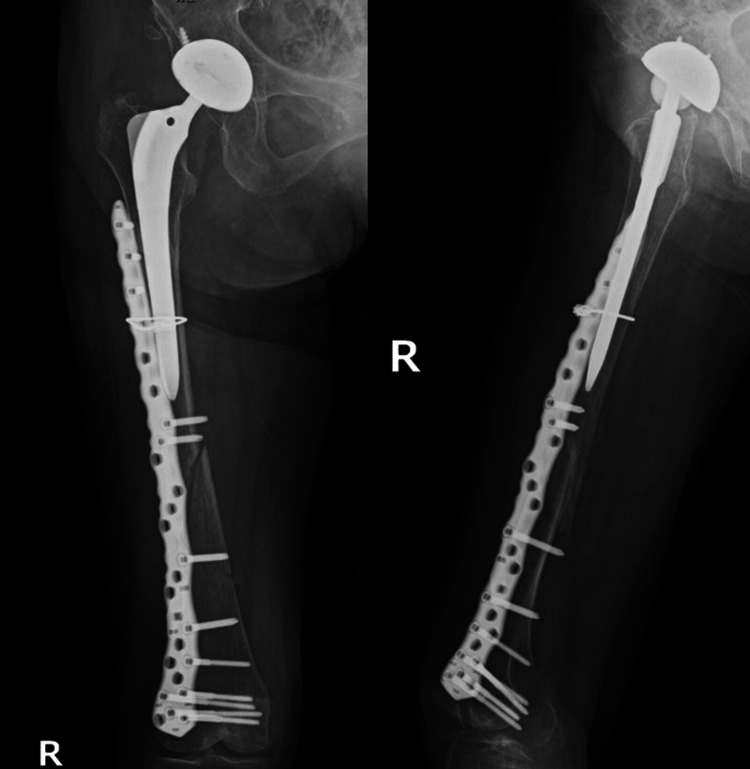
Postoperative radiographs of case 4 ORIF with plate fixation and cerclage wiring was performed. ORIF: open reduction and internal fixation.
